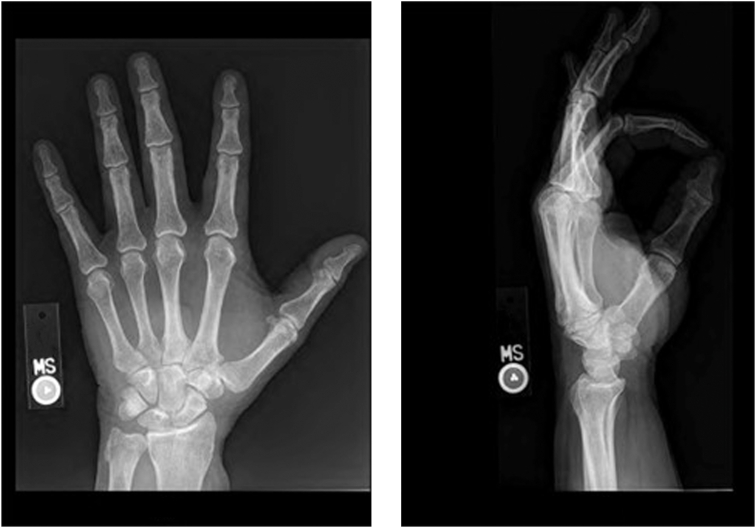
Anteroposterior and lateral left hand radiographs showing minimal degenerative arthritis.
